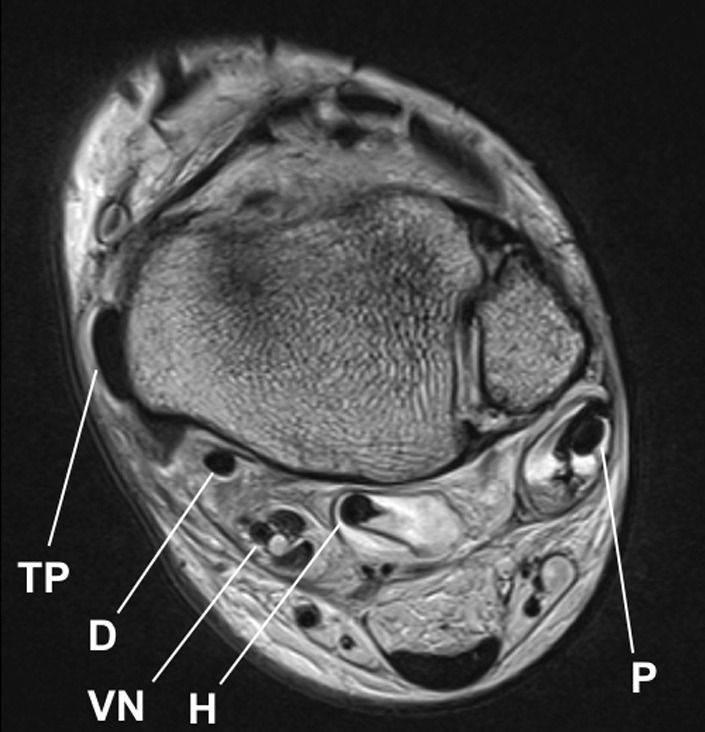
MRI showing the posterior tibial tendon on top of the medial malleolus. *TP* tibial posterior tendon, *D* flexor digitorum tendon, *VN* neurovascular bundle, *H* flexor hallucis longus tendon, *P* long peroneal tendon
